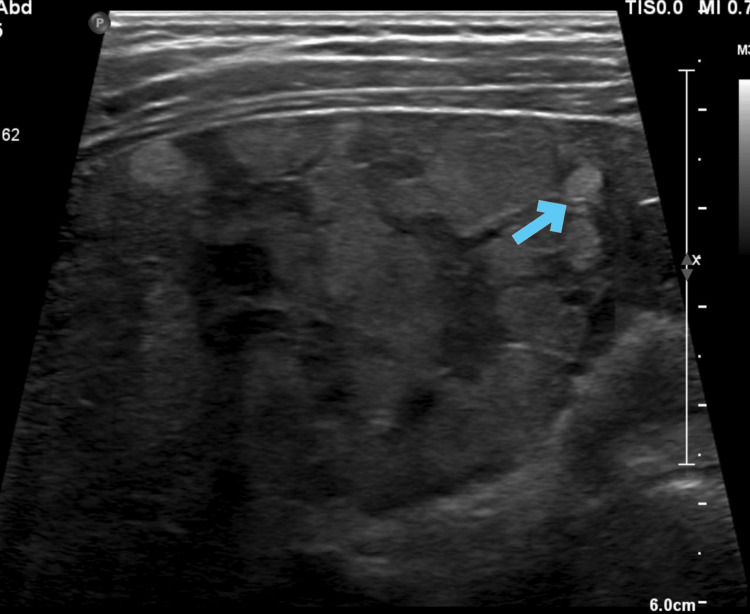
Ultrasonography of the abdomen of the youngest sibling The image shows features of chronic liver disease with regenerating nodules (blue arrow)
